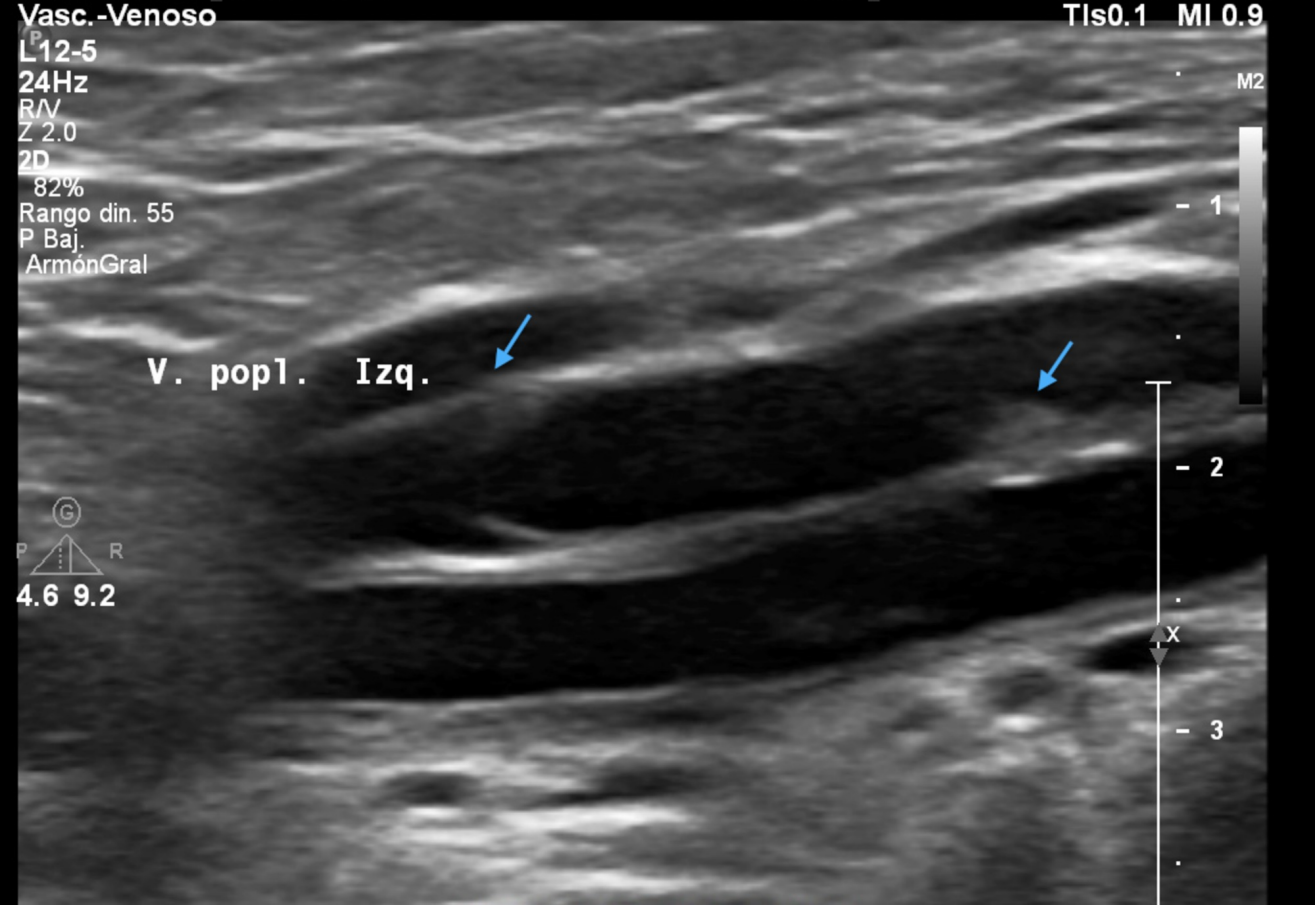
Deep vein thrombosis of the left popliteal vein Sonogram of the left popliteal vein (V. popl. Izq.) showing thrombi (blue arrows).
